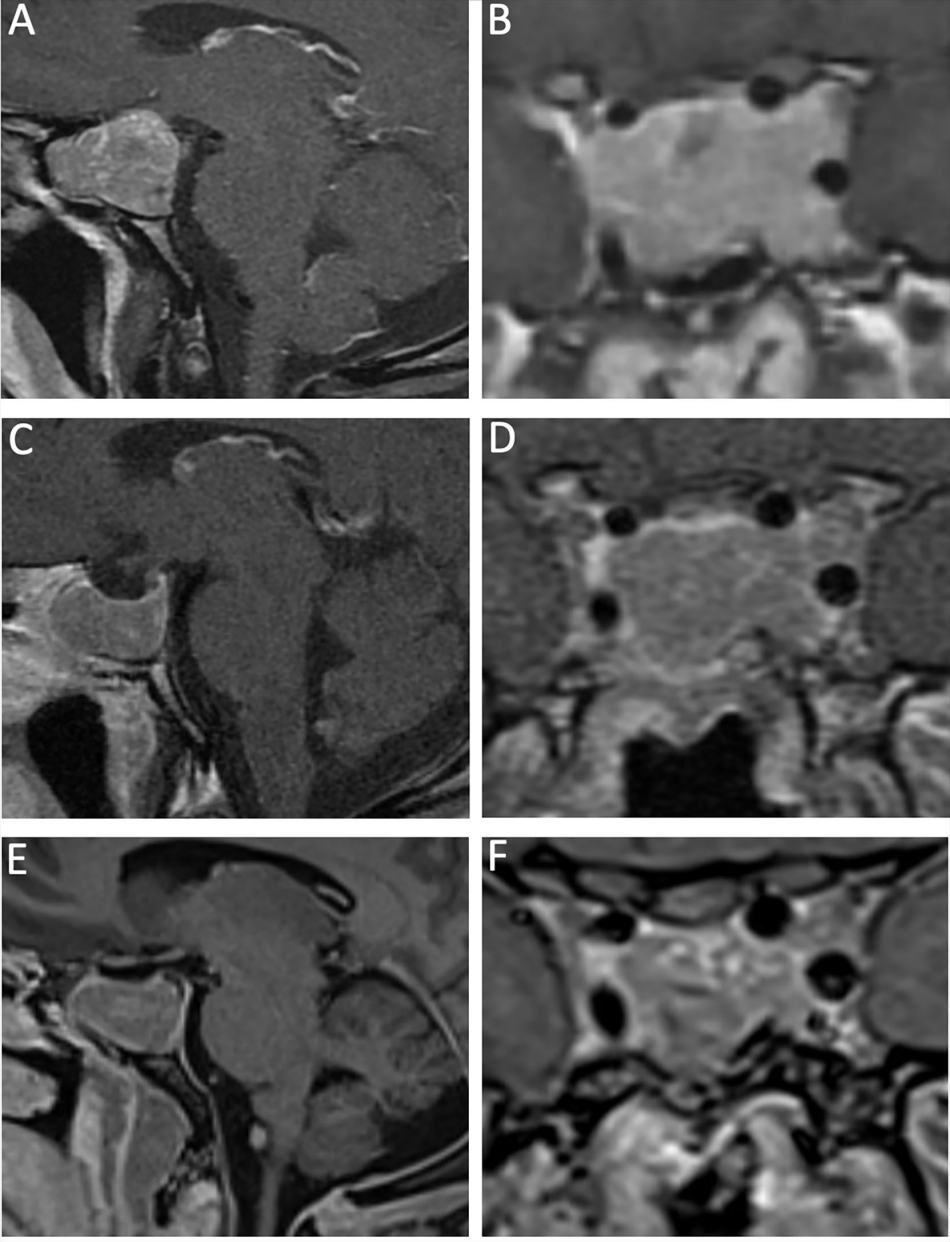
A 40-year-old man with a recurrent GH-secreting macroadenoma. His first surgery was completed at an outside institution. Preoperative MRI, T1-post gadolinium contrast, featuring sagittal (A) and coronal (B) views that show a macroadenoma with bilateral Knosp 3 invasion into the cavernous sinuses. 3-month postoperative MRI, T1-post gadolinium contrast, sagittal (C) and coronal (D) views show radical resection of the lesion, with very small residual in the left Knosp 3A compartment. More than 1 year from surgery, there is stable residual tumor in the left cavernous sinus as demonstrated by the sagittal (E) and coronal (F) MRI T1-post gadolinium contrast. The patient achieved biochemical remission with lanreotide.
